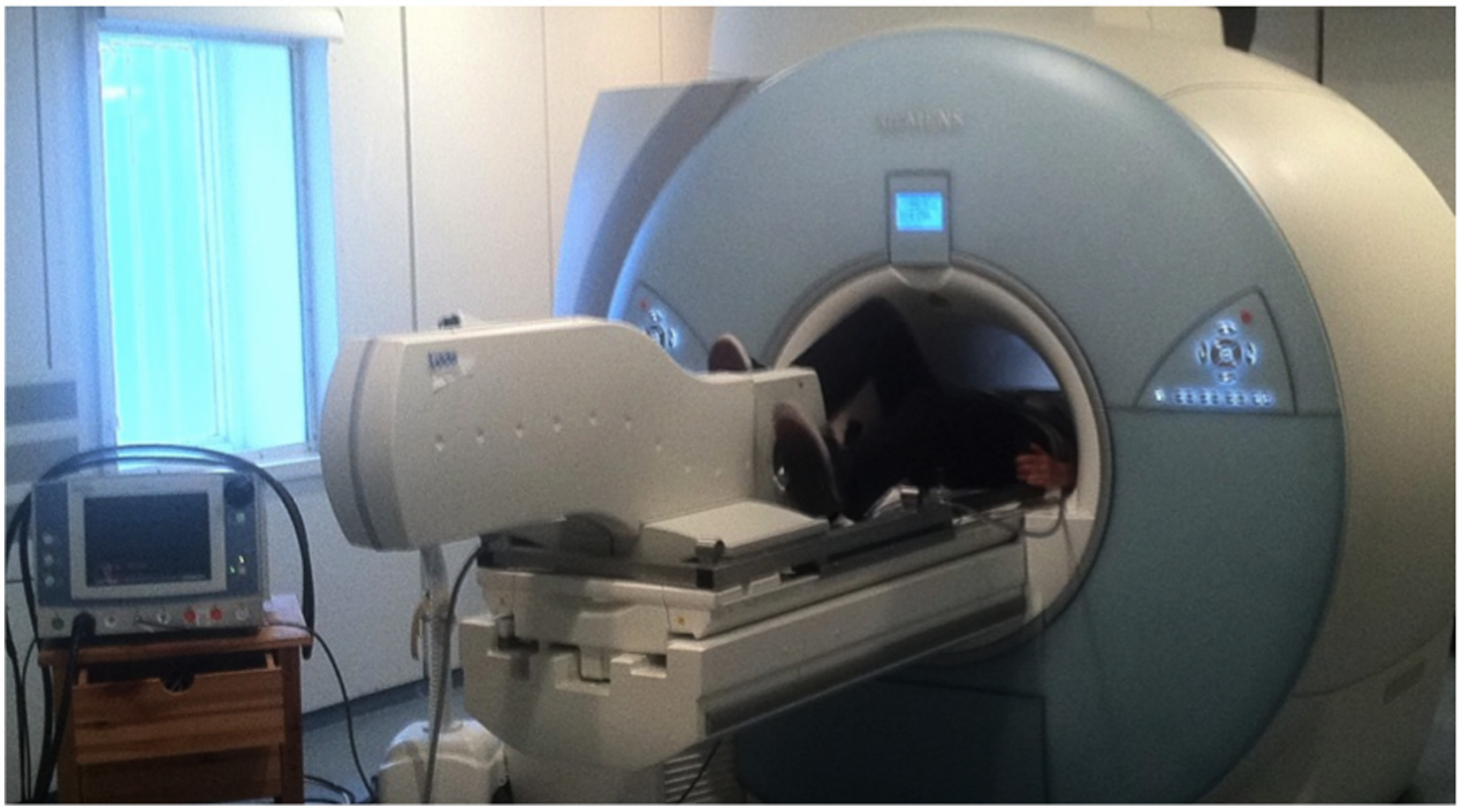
Amagnetic ergometer (LODE, Netherlands) allowing a supine treadmill test during MRI (1.5 T).
